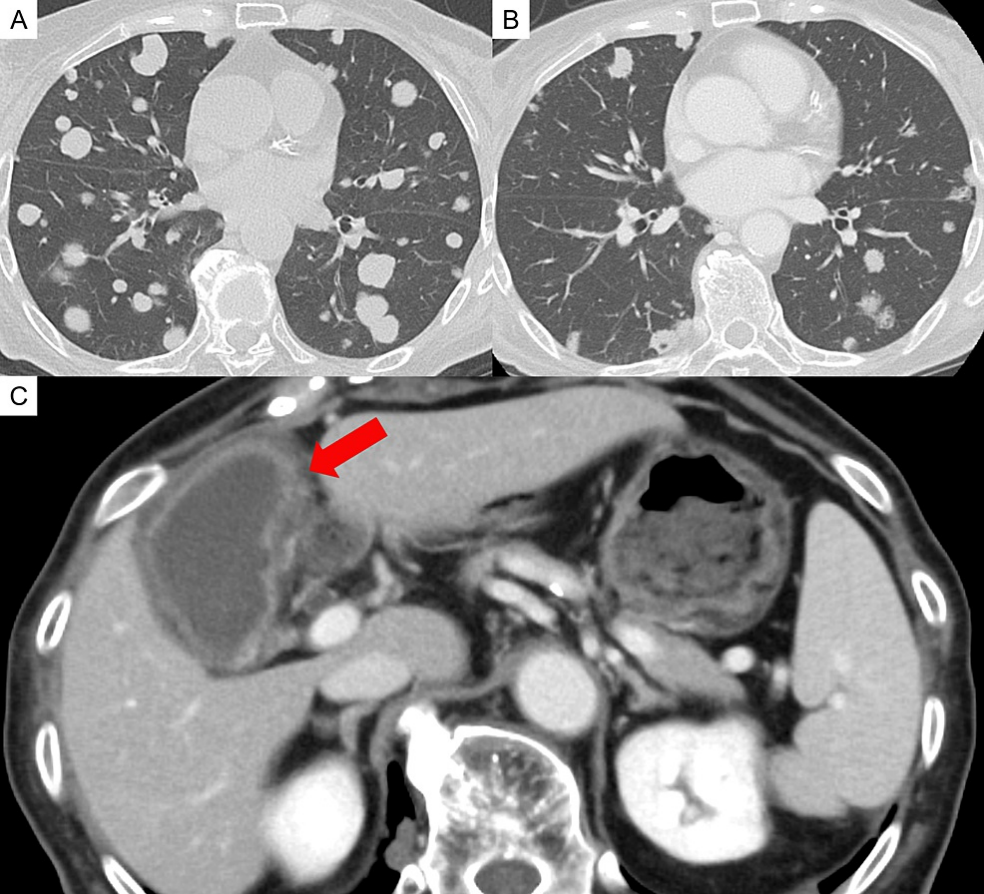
Computed tomography (CT) images at initial examination and after one month of treatment with lenvatinib. A) Computed tomography (CT) images of the lungs at the initial examination; B) CT images of the lungs after one month of treatment with lenvatinib; and C) CT images of the abdomen after one month of treatment with lenvatinib. Lenvatinib treatment reduced lung metastases (A and B) but caused edema around the gallbladder (arrow in C).
